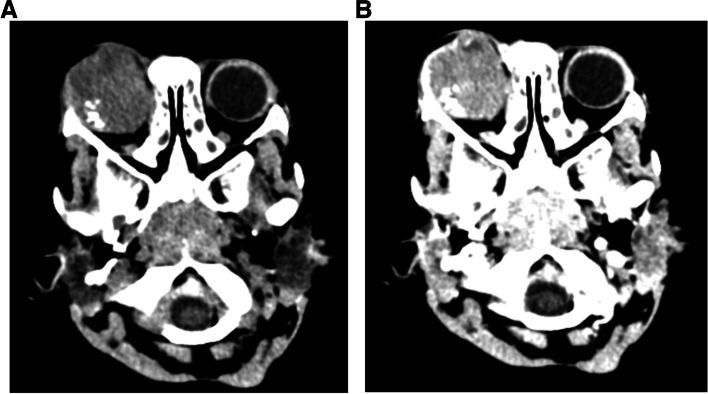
A An unenhanced axial CT at the level of the orbits. There was a large right intra-ocular mass, with calcifications, involving the anterior and posterior chambers. The mass measures 3.7 × 3.4 cm. B A contrast enhanced CT and demonstrated the same features as on A. There was no significant enhancement of the right ocular mass and the calcifications were visible. A diagnosis of right orbital retinoblastoma was made on A and B
